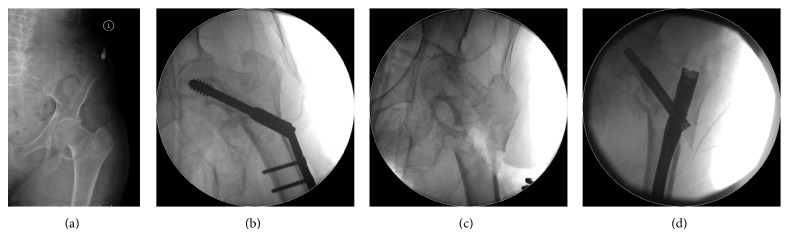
(a) 95-year-old female with proximal femoral fracture (AO/OTA type 31-A3). (b) Intraoperative radiograph showing the DHS. (c) Intraoperative radiograph after removal of the DHS. (d) Intraoperative radiograph showing the revision with AFFIXUS nail.
